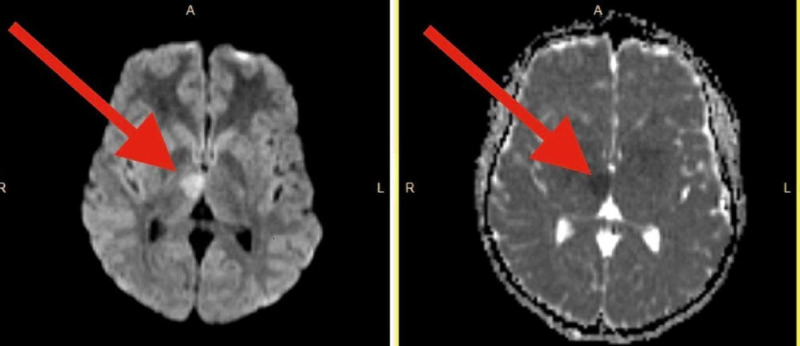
Brain MRI: right thalamic infarct with a small penumbra on diffusion weighted imaging and apparent diffusion coefficient mapping
